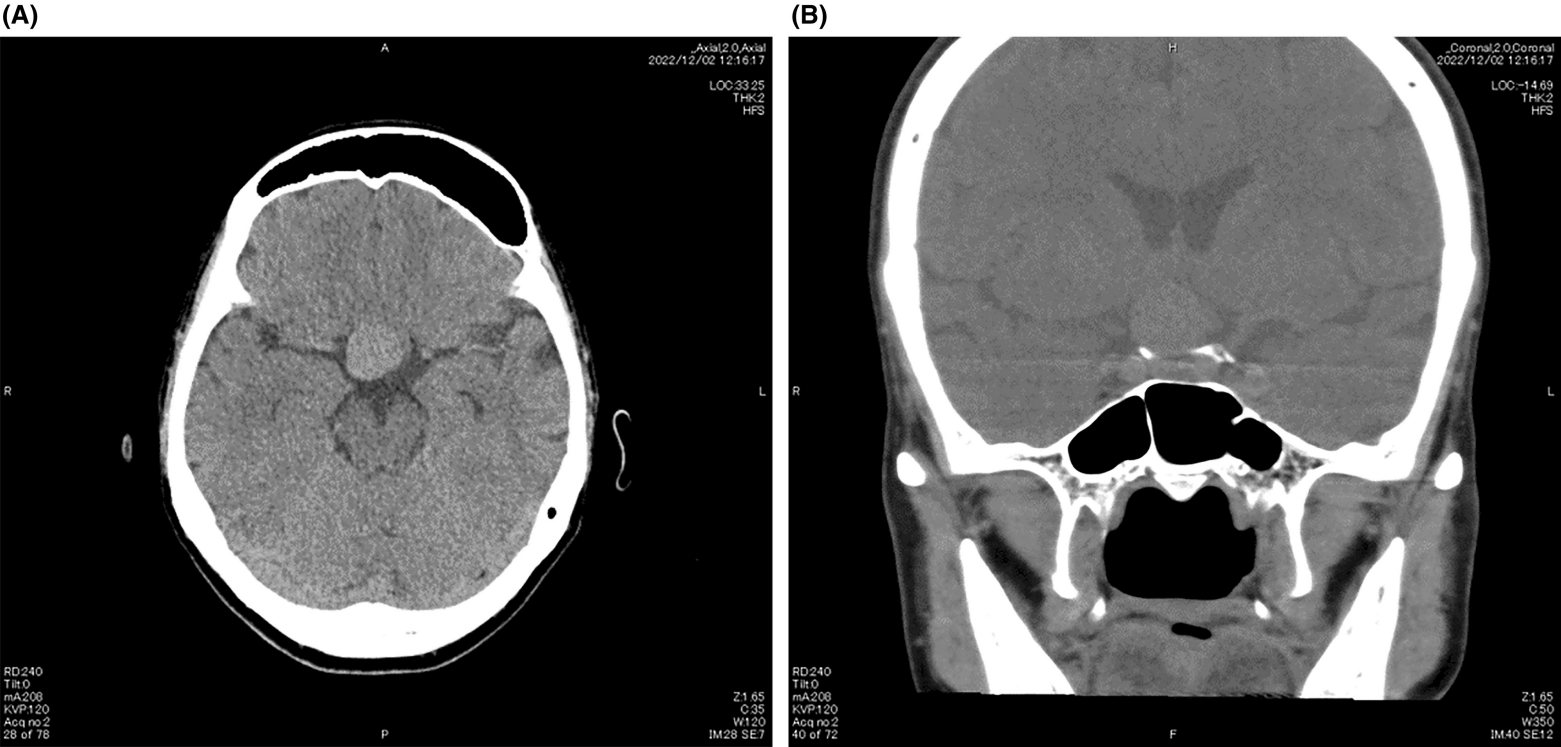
Brain computed tomography findings. (A) Axial and (B) sagittal images showing a large mass slightly to the right above the sella.
